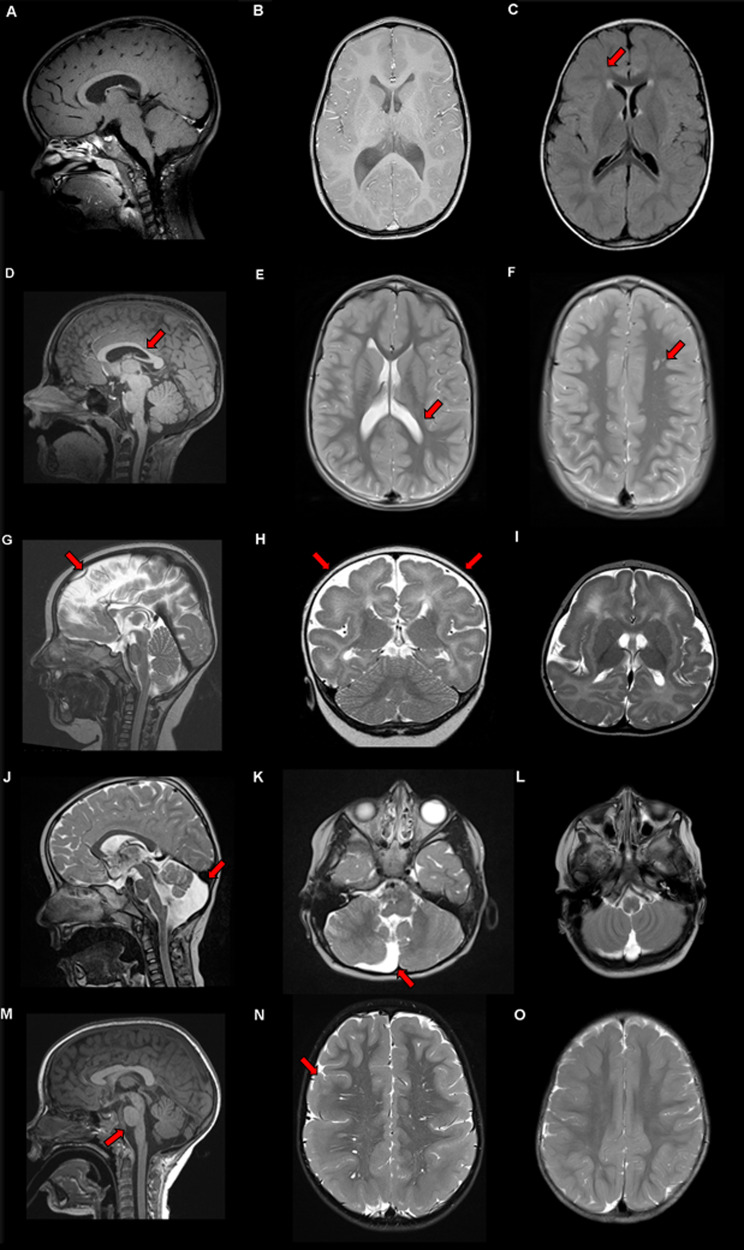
Brain abnormalities found in patients with mutations in *DYNC1H1*. The arrow in the figure points out the brain abnormality described in the figure legend. a Sagittal and b axial T1 weighted image of P6 at the age of 3 years showing normal brain anatomy. c Axial FLAIR sequences in P5 at 23 months-of-age showing periventricular lesions and cortical atrophy. d Sagittal T1 weighted MRI of P9 at the age of 7 years, revealed thinning of posterior body of corpus callosum. e Axial T2-weighted MRI of P9, revealed enlarged posterior horns of lateral ventricles and periatrial white matter volume loss. f Axial T2-weighted MRI of P9, indicating cortical heterotopia in left frontal deep white matter. g Sagittal, h coronal, and i axial T2-weighted MRI of P4 at the age of 7 months-of-age showing diffuse pachygyria with cortical thickness >10 mm with frontal and perisylvian predominance. j Sagittal and k transversal T2-weighted MRI of P7 at the age of 4 years showing an isolated dysplasia of the right cerebellum and cerebellar vermis with an enlargement of the fourth ventricle on the left and notably without the involvement of the pons or mesencephalon. l Transversal T2-weighted MRI of P5 at 23 months-of-age showing in contrast to k no vermis or ventricle enlargement. m Sagittal T2-weighted MRI of P10 at the age of 4 years showing brain stem hypoplasia. n Axial T2-weighted MRI of P10 showing bilateral temporal and parietal pachygyria reaching until dorsal part of the frontal lobe. o Axial T2-weighted MRI of P5 at 23 months-of-age showing cortical brain atrophy without pahygyria
